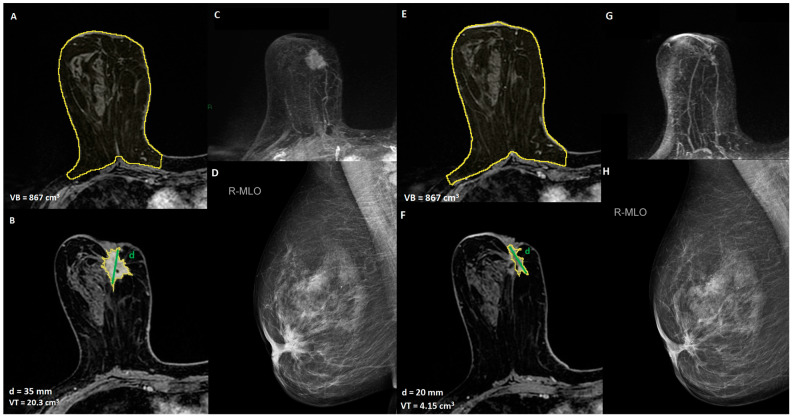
A 52-year-old women with unifocal HER2 positive invasive ductal carcinoma, infiltrating the nipple areola complex, with indication of breast-conservative surgery after NACT: (A,B) T1-weighted post-contrast MRI to define the extension of the disease and breast volume, VB = breast volume, VT = tumor volume, d = diameter of the lesion; (C) maximum intensity projection (MIP) pre-NACT image; (D) mediolateral oblique mammogram of the lesion, (E,F) T1-weighted post-contrast MRI to assess the response to NACT, (G) maximum intensity projection (MIP) post-NACT image, (H) mediolateral oblique mammogram of the lesion post-NACT.
